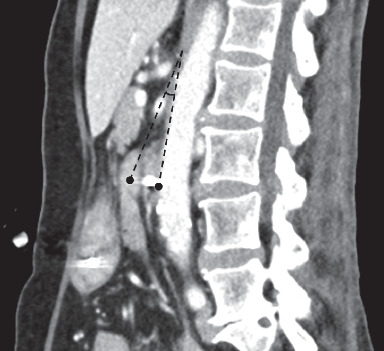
Sagittal multiplanar reconstructed CT scan image of the aortomesenteric angle (black dashed line) of approximately 23 degrees.
